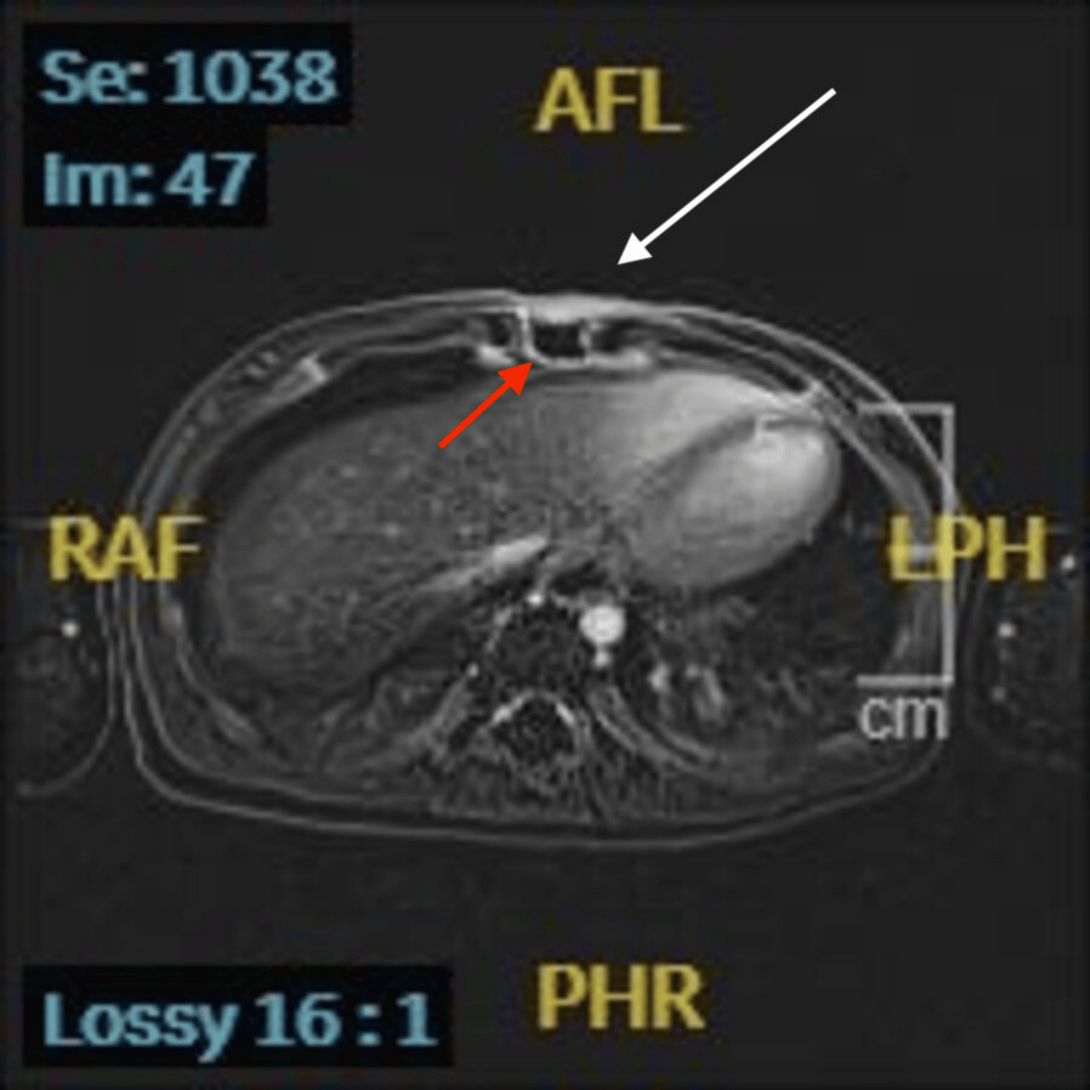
Contrast-enhanced axial T1-weighted MRI demonstrating subcutaneous swelling and enhancement (white arrow) at the xiphoid level (red arrow), consistent with cellulitis.
